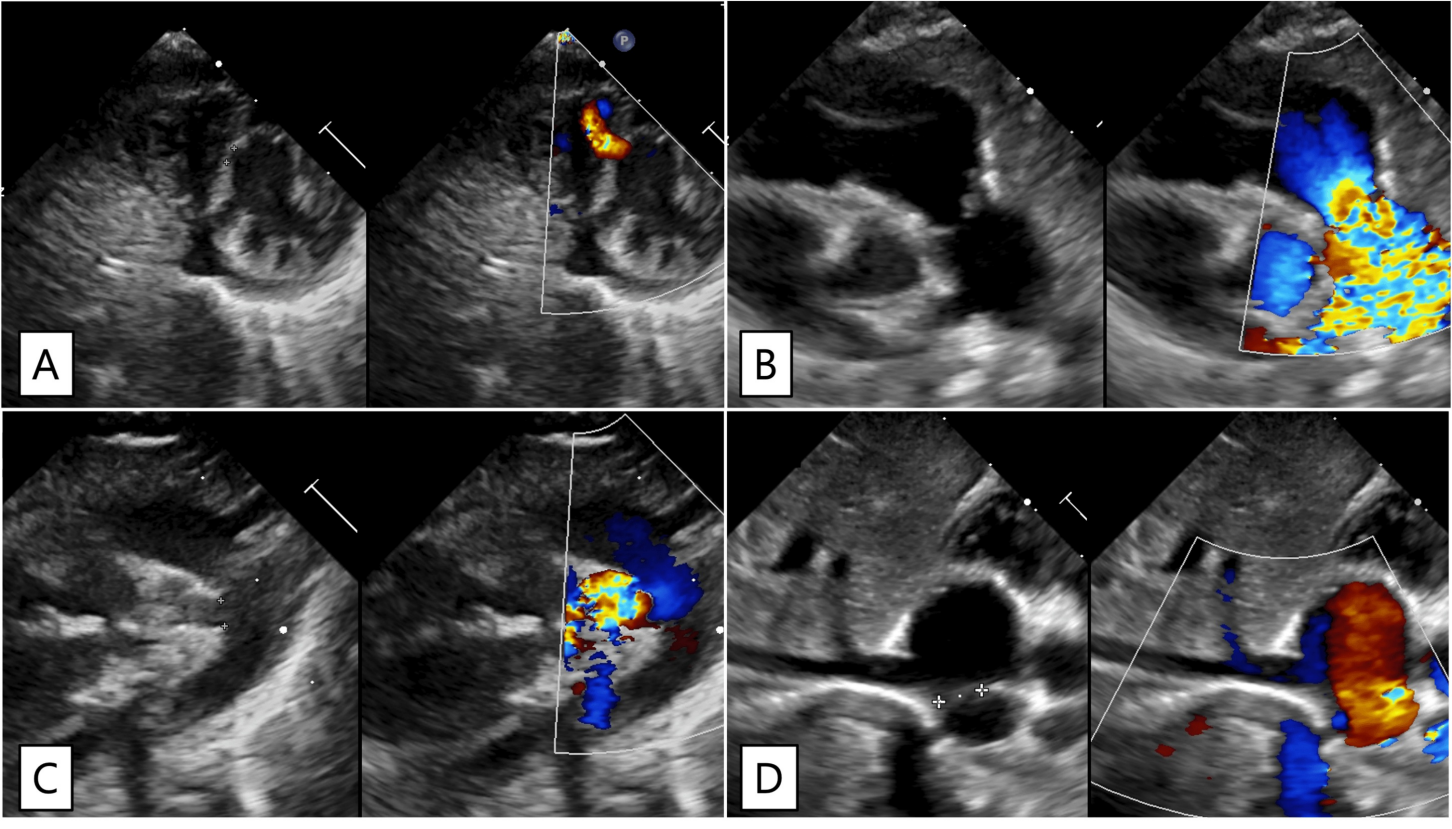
Echocardiographic findings. A: Muscular ventricular septal defect. Left-to-right shunting can be observed in the muscular portion of the interventricular septum; B: Valvular pulmonary stenosis. Post-stenotic doming of the pulmonary artery can be observed. The peak flow velocity in the main pulmonary artery is 3.6 m/s, corresponding to a pressure gradient of 52 mmHg; C: Patent ductus arteriosus. The diameter of the ductus arteriosus is 2.2 mm, with left-to-right shunting observed; D: Atrial septal defect (ASD). Left-to-right shunting can be observed at the atrial level. The size of the ASD was approximately 7 mm.
